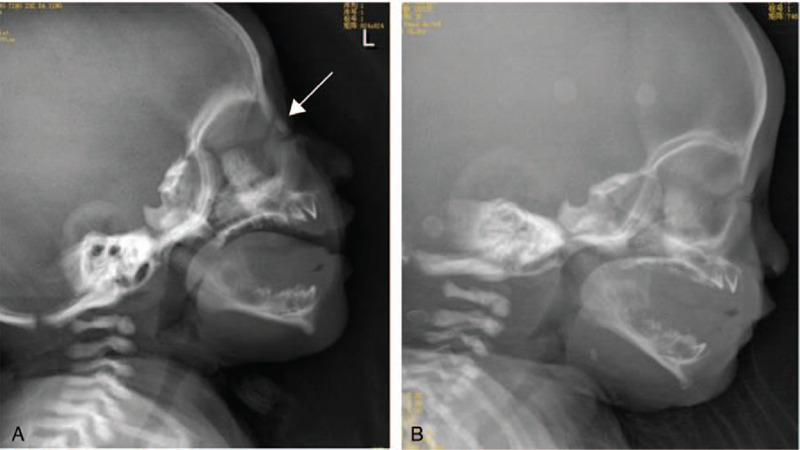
Radiograph shows normal nasal bone in the newborn twin A (arrow) (A) and absence of the nasal bone in newborn twin B (B) on postnatal day 3.
